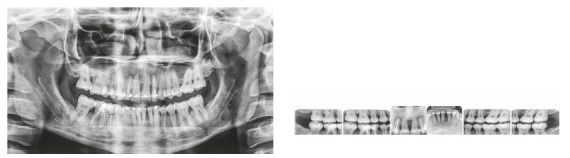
Retention radiographs of Case 1.
